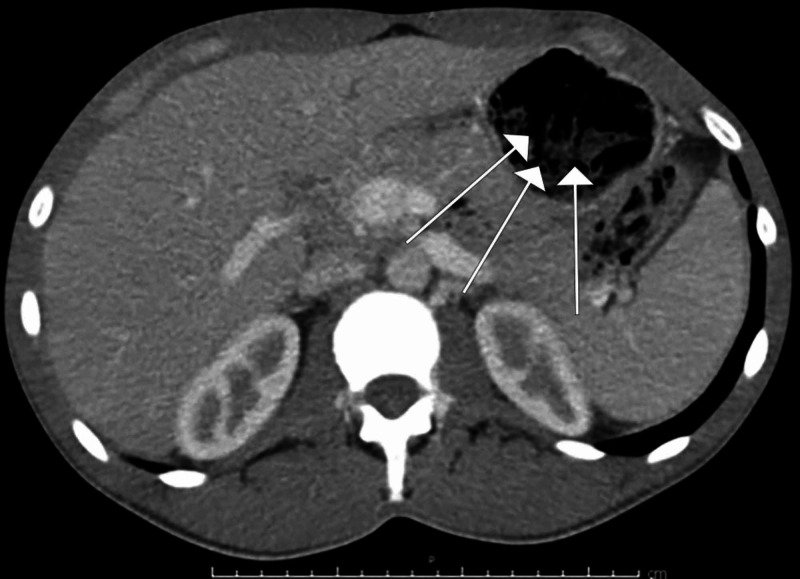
Computed tomography (CT) axial tomogram demonstrating amorphous material in the stomach compatible with ingested plastic bags (marked with white arrows).
